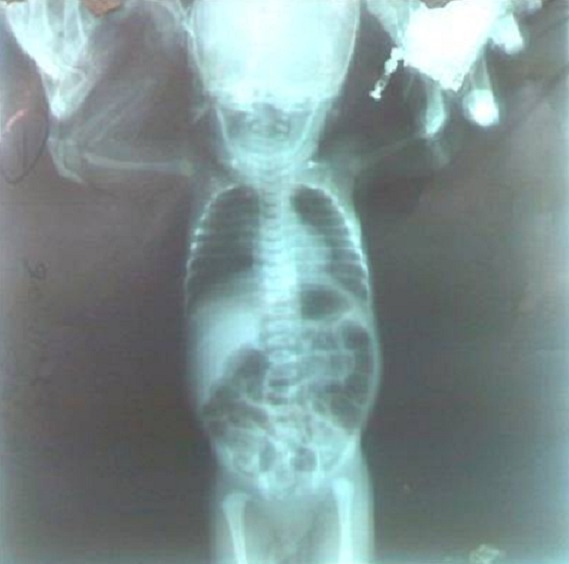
Radiographie thoraco-abdominale sans préparation réalisée au 2ième jour a révélé un enroulement de la sonde au niveau de la 4ième vertèbre cervicale et de nombreuxgaz au niveau des intestins
